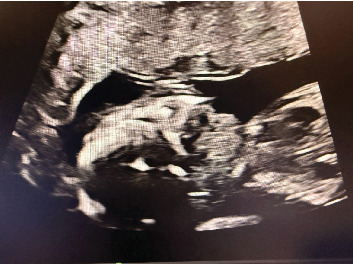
Imaging findings. 2D ultrasound of the fetus at 20 weeks gestation, showing an anterior fetal neck mass.
